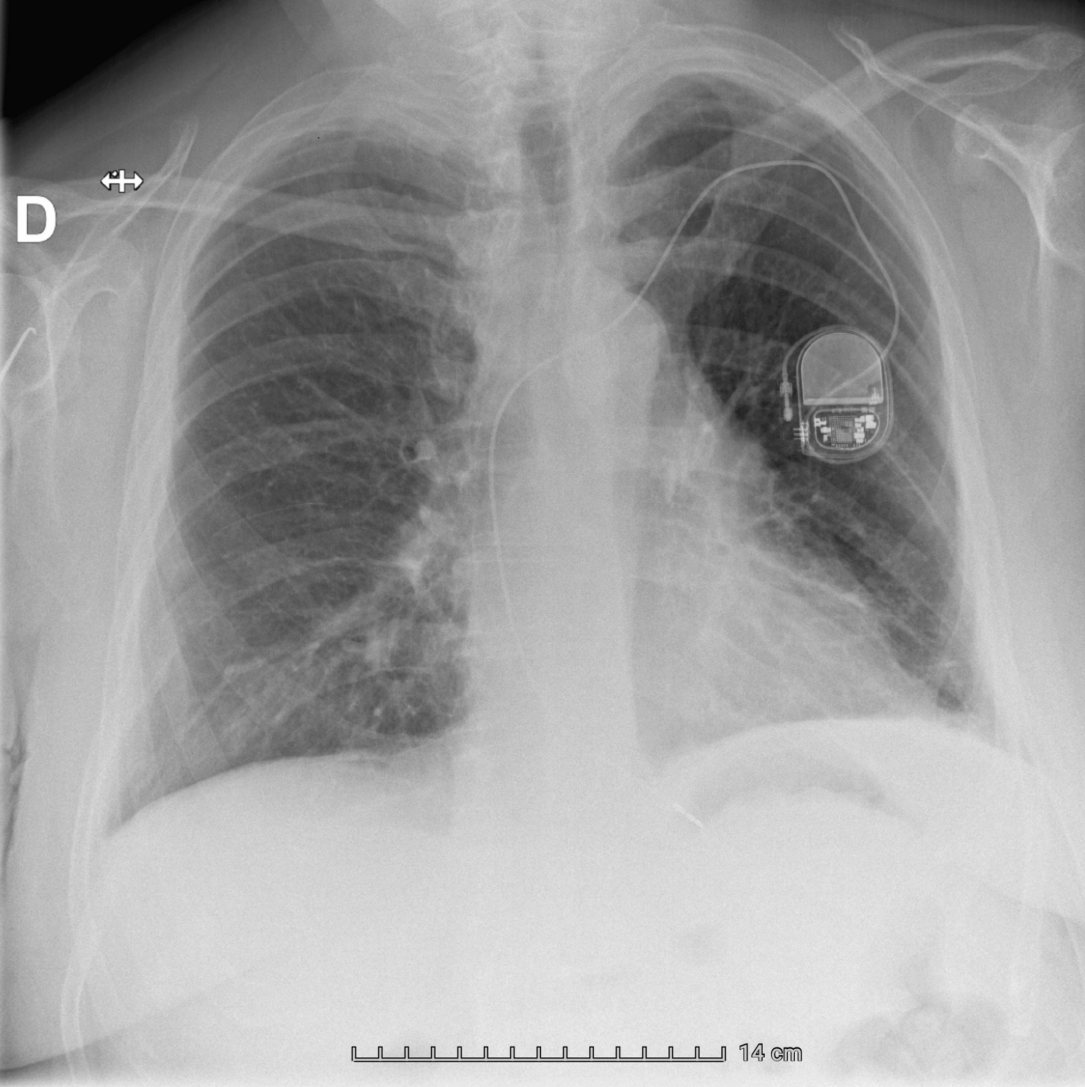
Control chest X-ray on postoperative day one.
